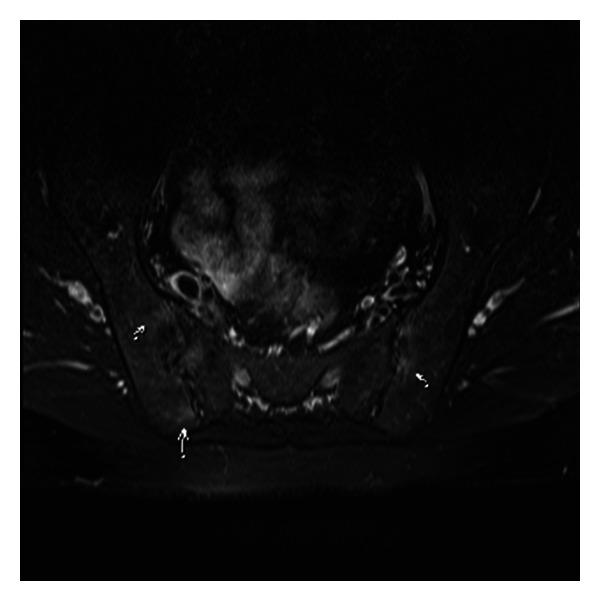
MRI of patient with KS showed active sacroiliitis.
